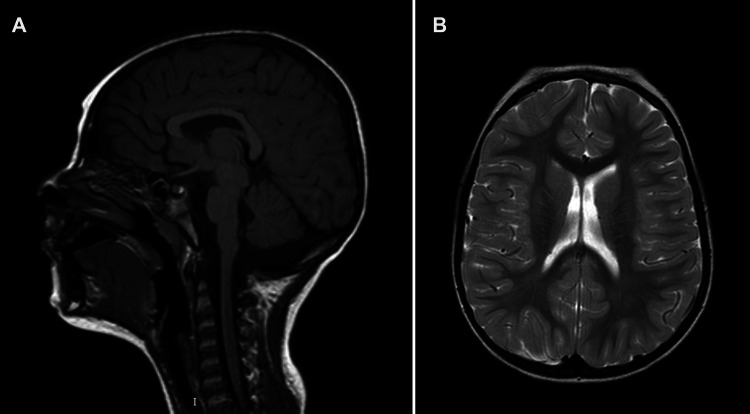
Brain MRI of patient 2 at 3 years of age. (A) Mid-sagittal T1-weighted image showing hypoplasia of the corpus callosum. (B) Axial T2-weighted image at the level of the lateral ventricles.
